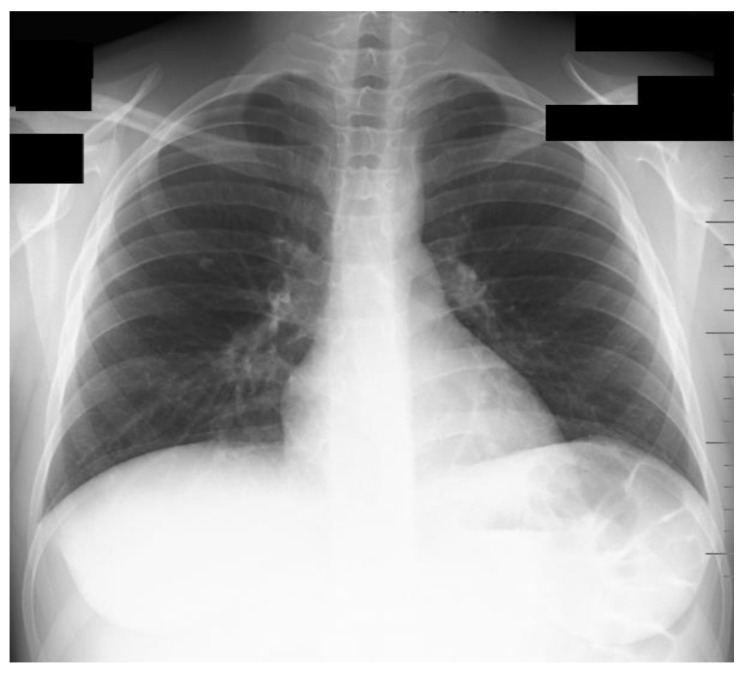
Chest X-ray before surgery (anteroposterior view).
